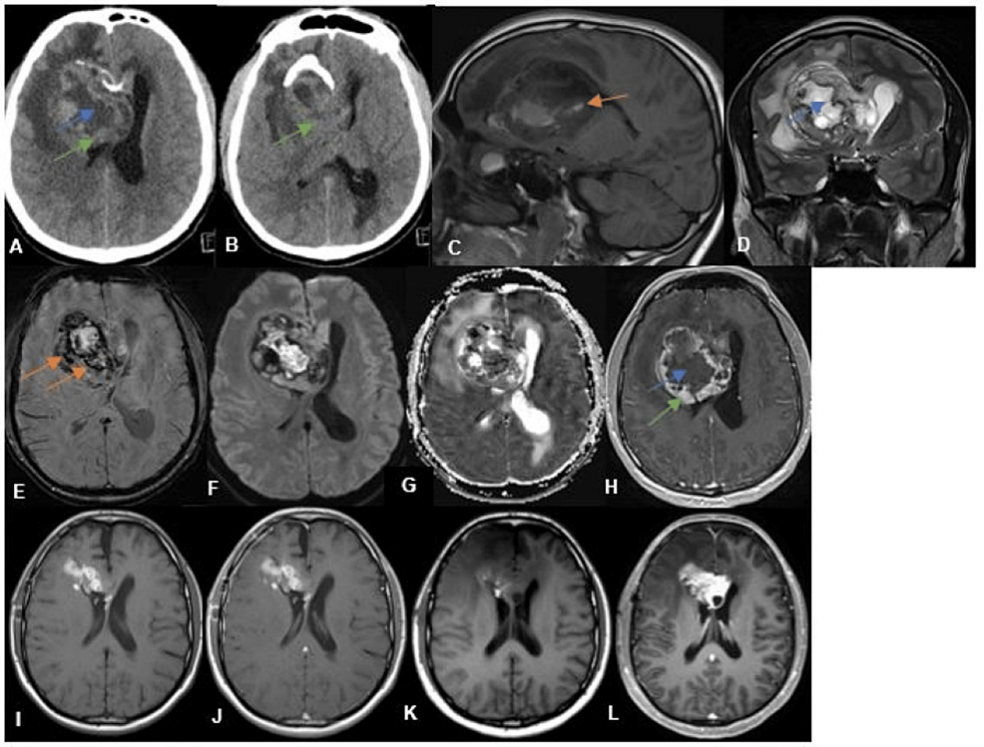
CT and MRI findings (A, B) CT scan showed an apparently intraventricular mass lesion centered in the right lateral ventricle. Brain MRI sagittal T1 (C), coronal T2 (D), SWI (E), DWI (F), ADC map (G), and T1 post-contrast (H) showed a heterogeneous right intraventricular mass lesion expanding the frontal horn and body. The lesion was mostly hypointense on T1, hyperintense on T2 with cystic and necrotic areas (blue arrows), hemorrhagic components (orange arrows), and a peripheral solid component (green arrows), with areas of restricted diffusion and post-contrast enhancement of the solid component. Additionally, perilesional edema and hydrocephalus were observed. Post-surgery MRI axial T1 (I) and T1 post-contrast (J) showed tumor resection and post-surgery subacute blood products. Brain MRI axial T1 (K) and T1 post-contrast (L) seven months after surgery demonstrated tumor recurrence CT: computed tomography; MRI: magnetic resonance imaging
